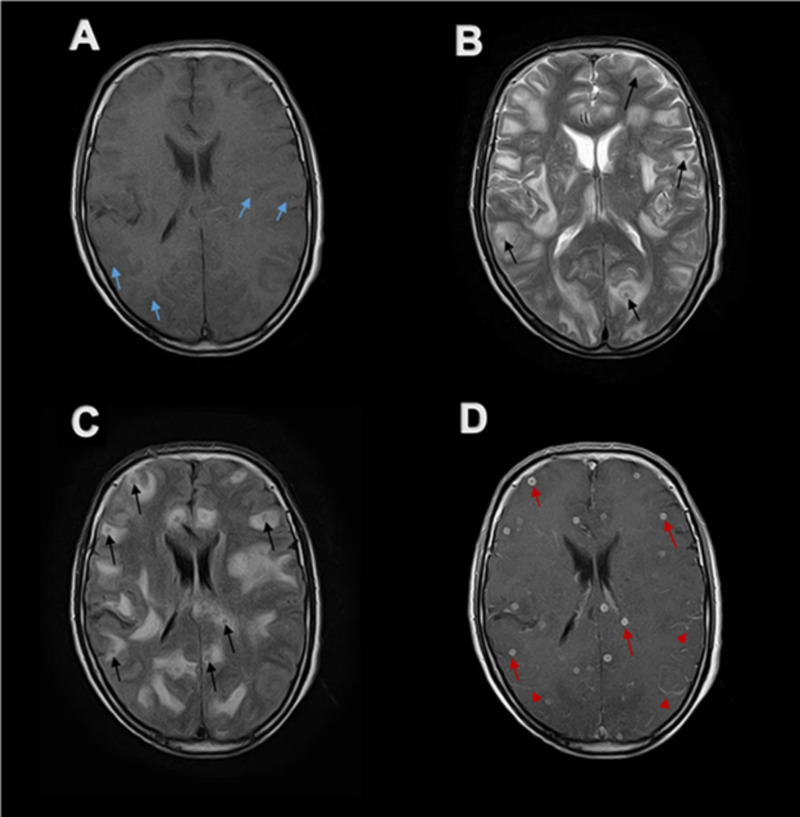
Axial MR imaging of brain at the level of basal ganglia Multiple small lesions are distributed in shower like pattern throughout the brain involving all lobes bilaterally with extensive perilesional edema. (A) Lesions appear as isointense to hypo-intense on axial T1-weighted MR image (blue arrows). (B and C) Same lesions appear hypo-intense on axial T2-weighted and FLAIR MR images (black arrows). (D) Ring enhancement is shown on post-contrast axial T1-weighted MR image (red arrows) with associated patchy gyriform leptomeningeal enhancement (red arrow heads). MR, magnetic resonance. FLAIR, fluid-attenuated inversion recovery
